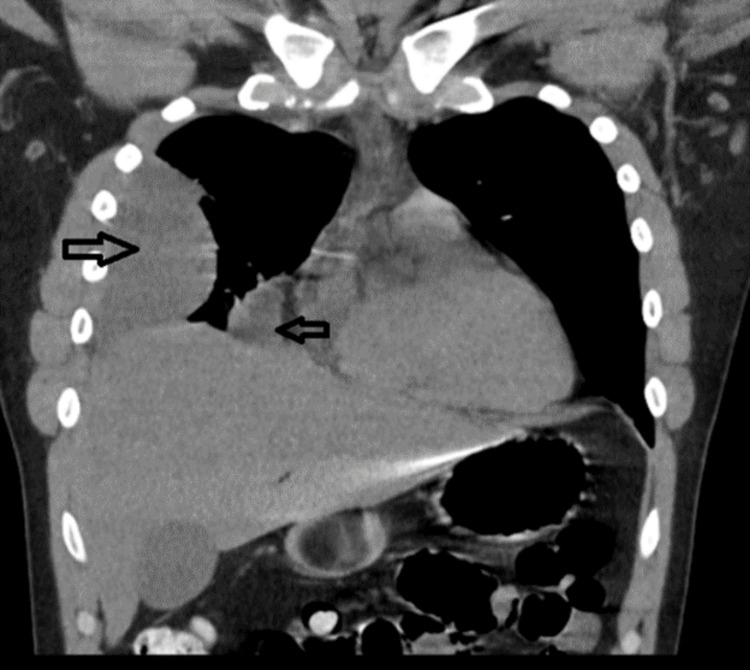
Repeat CT scan of chest showing reduced pleural effusion with anteriorly located loculated effusion (arrows)
